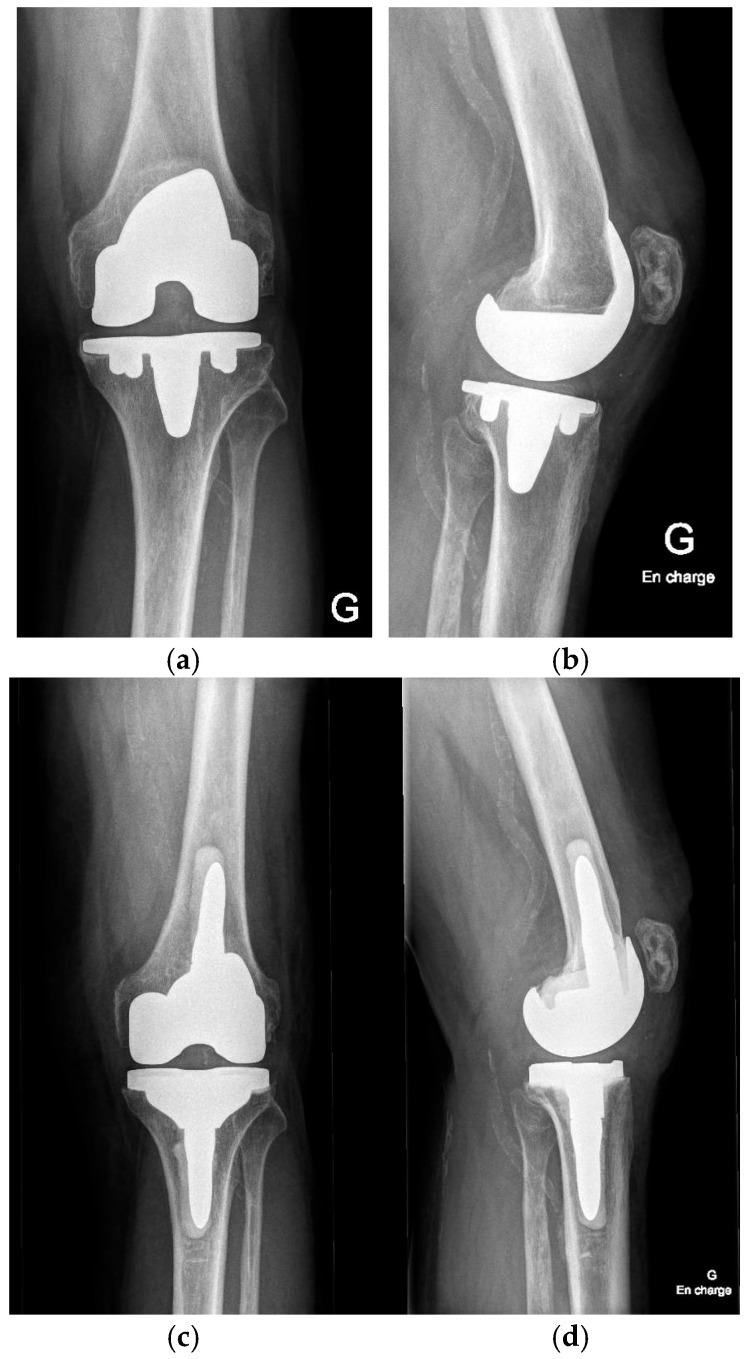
Radiographic comparison of preoperative (failed primary TKA due to aseptic loosening) and postoperative component positioning and alignment (a) preop AP view; (b) preop lateral view; (c) postop AP view; (d) postop lateral view.
